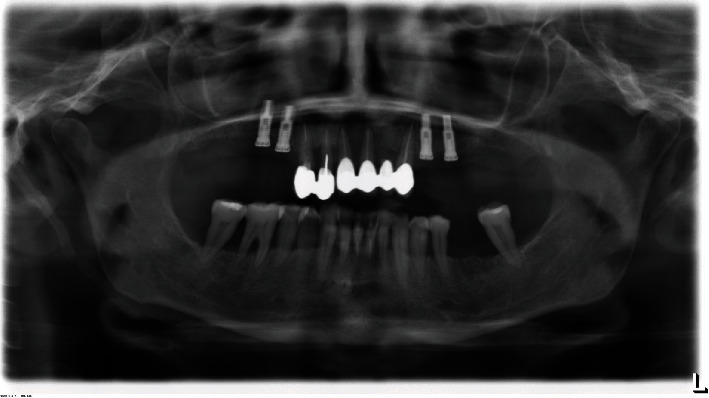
Orthopantomography performed in 2013.
